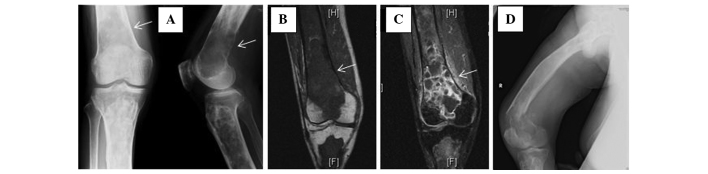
(A) Roentgenograms showing typical fibrous dysplasia involving the tibia and an ill-defined osteolytic lesion (arrow) in the right distal femur. (B) T1-weighted coronal-plane magnetic resonance imaging of the lesion of secondary osteosarcoma (arrow). (C) The lesion was intensively enhanced by injection of gadopentetate dimeglumine (arrow). (D) A pathological fracture through the lesion of the distal femur.
